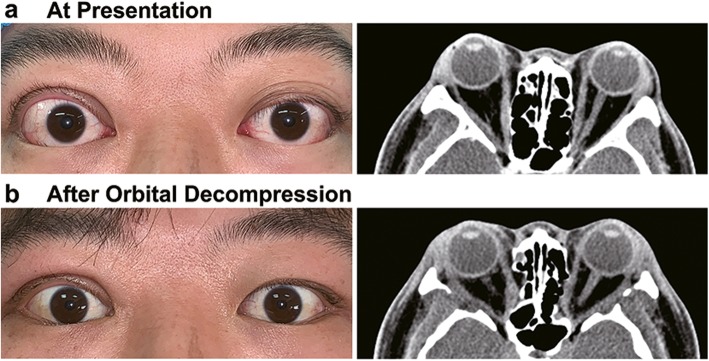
Case presentation (TAO with OMG). a A 32-year-old male complaint of bilateral proptosis for 2 years. Physical examination revealed slight ptosis of the left upper eyelid and mild retraction of the right upper eyelid. The patient reported a medical history of OMG, and the unnatural crease of the right eyelid resulted from previous blepharoplasty to correct ptosis. The CT scan showed bilateral enlargement of the medial rectus muscles. b Orbital decompression partially relieved the right upper eyelid retraction but resulted in new onset of left upper eyelid retraction due to increased motor impulses to sustain eyelid elevation. The CT scan showed balanced two-wall decompression in both orbits
